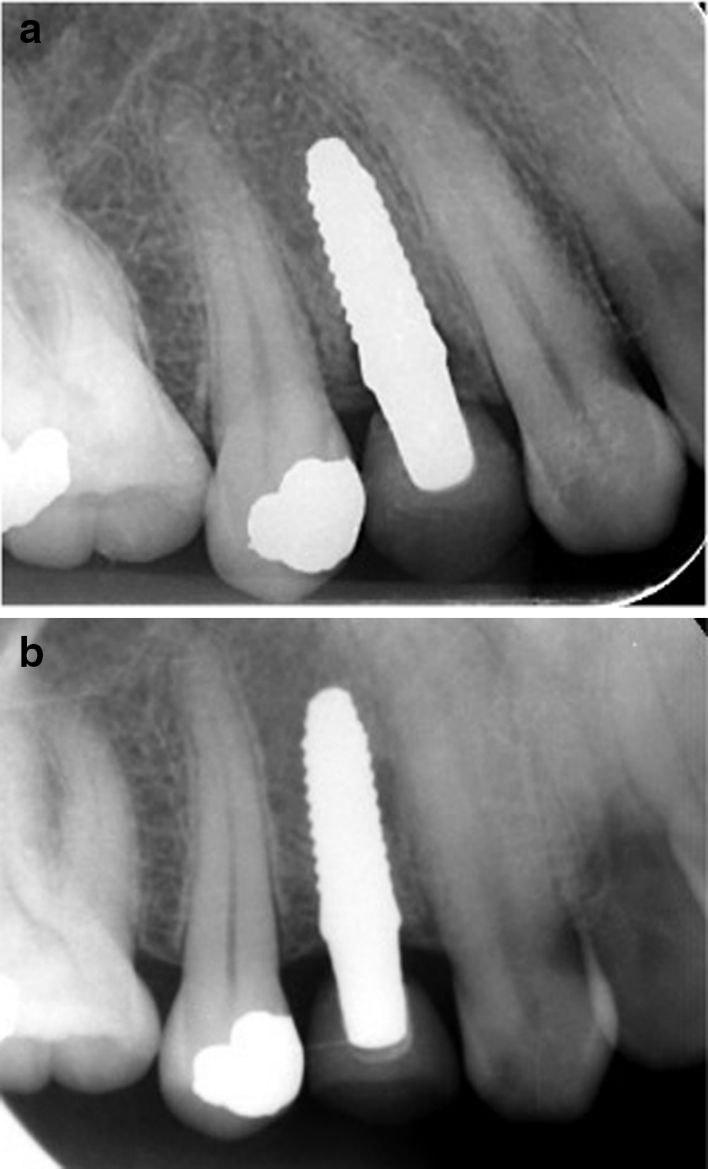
a Periapical radiographs of zirconia implant after 1 year. b Periapical radiographs of zirconia implant after 5 years
